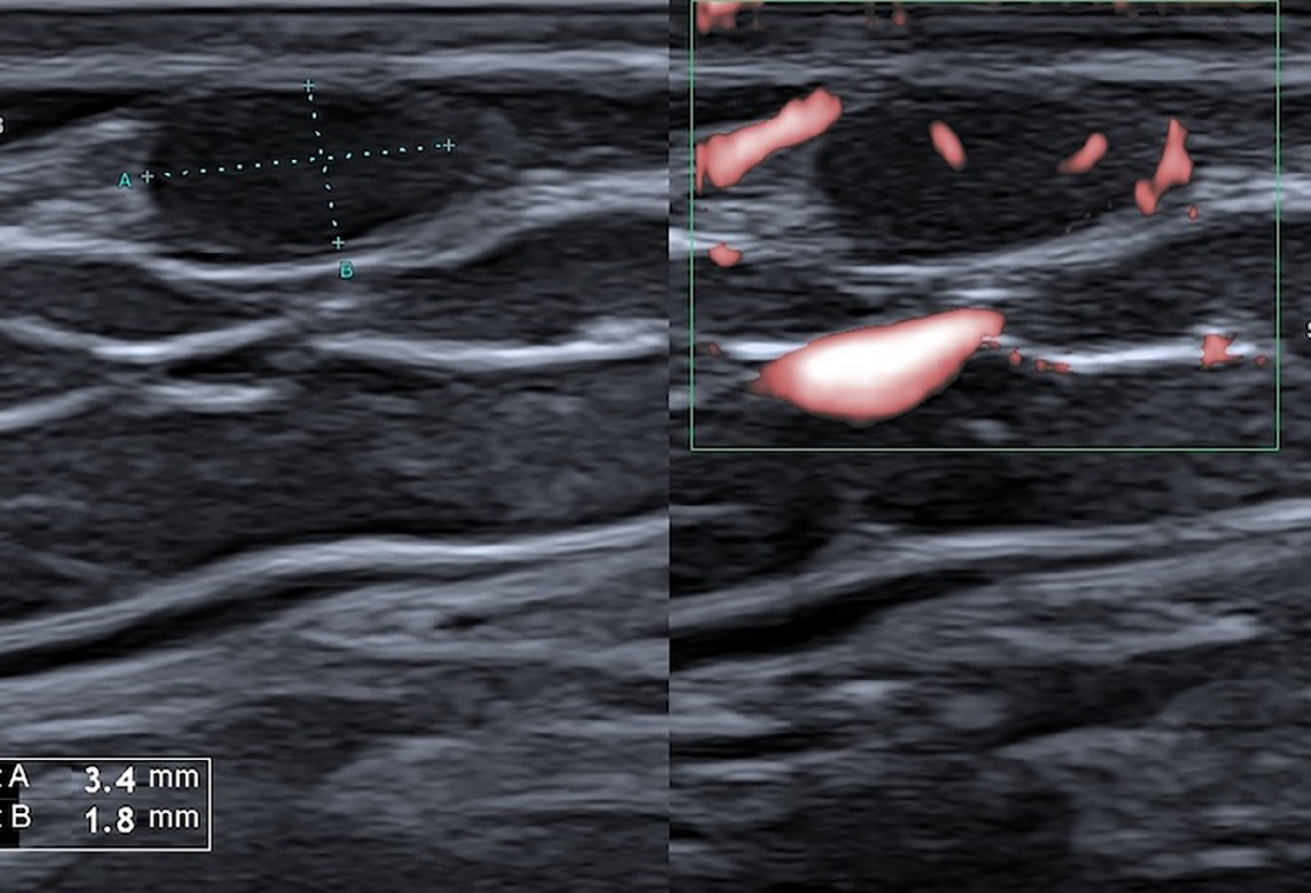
A 3‐mm large, in‐transit melanoma metastasis of the arm. B‐mode (left) and superb microvascular imaging scan (right). Ultrasound allows depicting the small, hypoechoic, subcutaneous nodule and demonstrating the presence of flow signals inside the metastasis.
